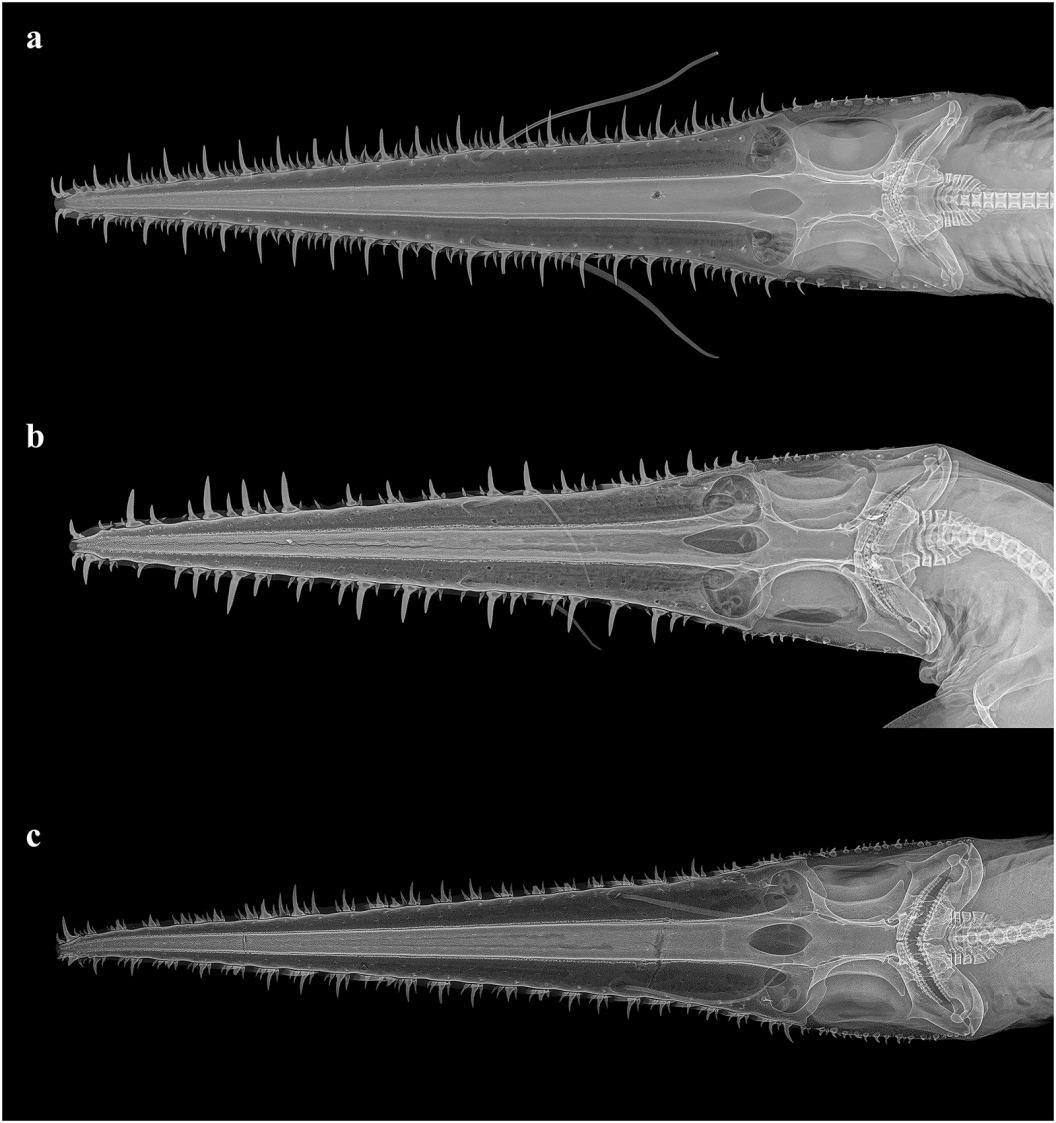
Radiographs of the crania of the three species of *Pliotrema* in dorsal views. a
